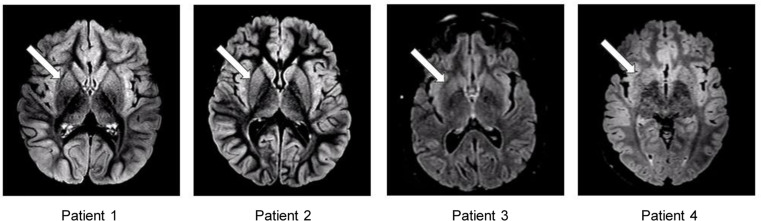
MRI analysis of BBB breakdown in individuals with ASLD. Comparison of neuroimaging findings among patients with ASLD. Abnormal hyperintensities are best seen on axial T2 FLAIR MRI for patient 1, patient 2, patient 3, and patient 4. Bilateral hyperintensities can be seen in the putamen, globus pallidus, heads of the caudate nuclei, and insular cortices. A gradation pattern (anterior to posterior) of hyperintensity can be seen in the bilateral putamen (arrow).
